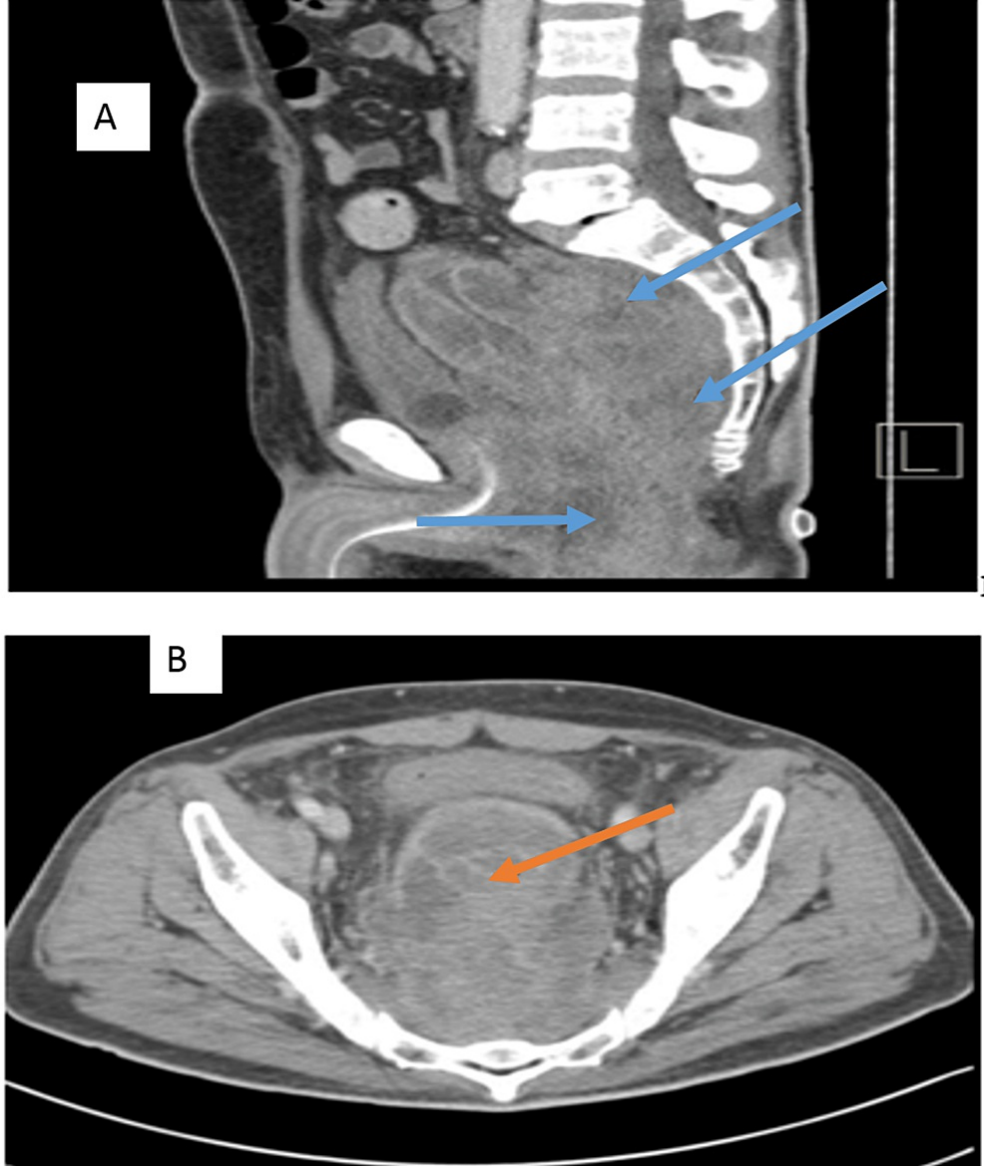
CT scan of the pelvis with intravenous contrast (Case 2) CT scan of the pelvis with intravenous contrast (A: sagittal plane, B: axial plane) demonstrates heterogeneously enhancing circumferential rectal mass (blue arrows) infiltrating the mesorectal fat and pre-sacral region (orange arrow), associated with pelvic fat stranding.
